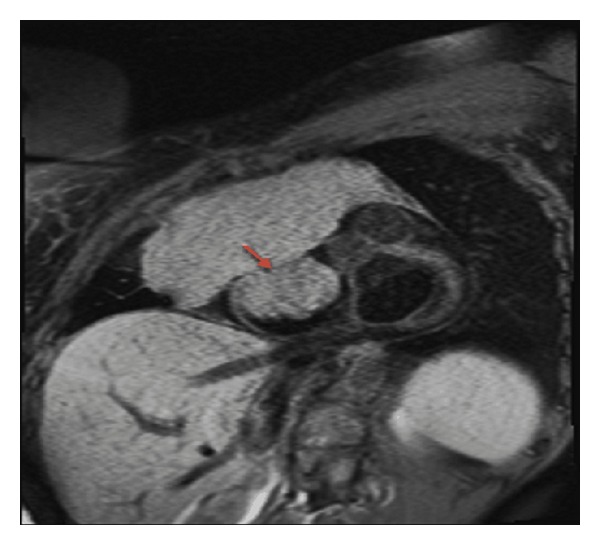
Cardiac MRI short axis T1 at the level of mitral valve reveals a large mediastinal mass infiltrating and obliterating the SVC causing SVC obstruction. The tumor extends into the right atrium (red arrow) and invades the tricuspid valve. Maximum diameter of the mediastinal mass measures 15 × 10 cm, and the mass in the right atrium measures 5.8 × 5.3 cm.
